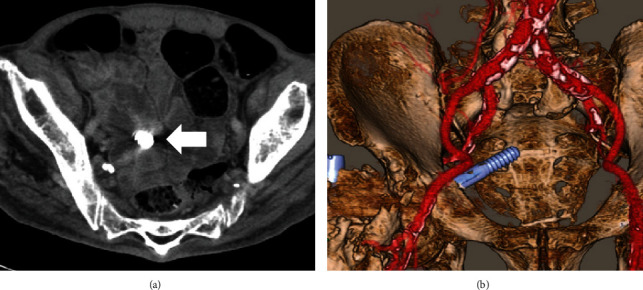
A contrast-enhanced computed tomography scan showing the lag screw located deep in the pelvis, between the internal and external iliac vessels, and tangent to the intestine. (a) Axial view (arrow, lag screw) and (b) reconstructed 3D image.
